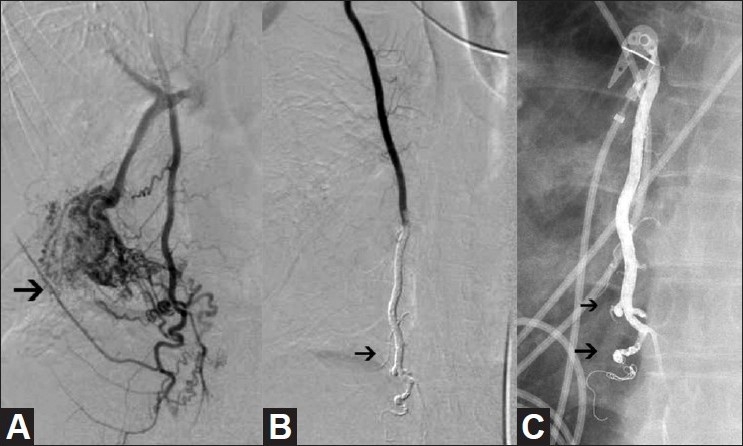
(A-C): Selective right internal mammary artery injection (A) shows the presence of a high-flow right internal mammary artery (IMA) to pulmonary artery malformation (arrow). Selective right IMA arteriogram (B) following coil occlusion of the IMA distal to the lowest contributory branch and Onyx injection shows cessation of flow to the malformation (arrow). Radiograph of the chest (C) the day after embolization shows penetration of Onyx (arrows) into the first-order branches of the IMA
